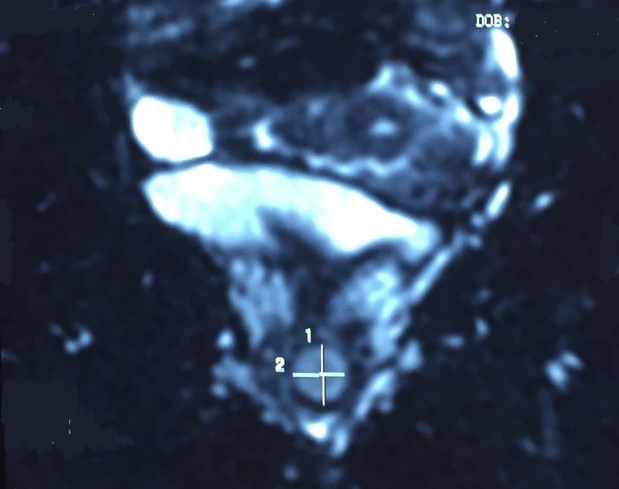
Coronal section of a pelvic MRI showing a urethral leiomyoma
